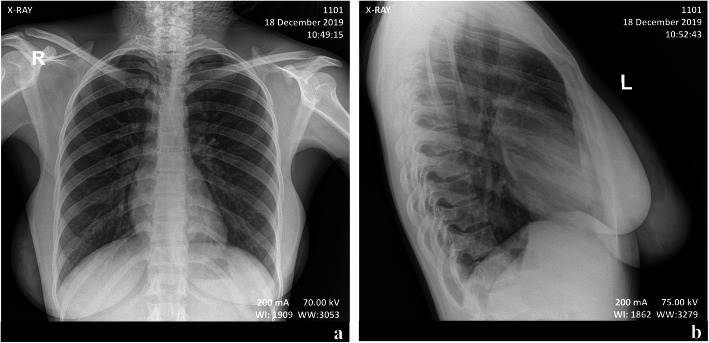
Chest X-rays of Patient 2. Subnormal chest X-rays of a 25-year-old female. Discrete peribronchovascular blur in the posterobasal regions. Normal aspect of the hila and the costophrenic angles
